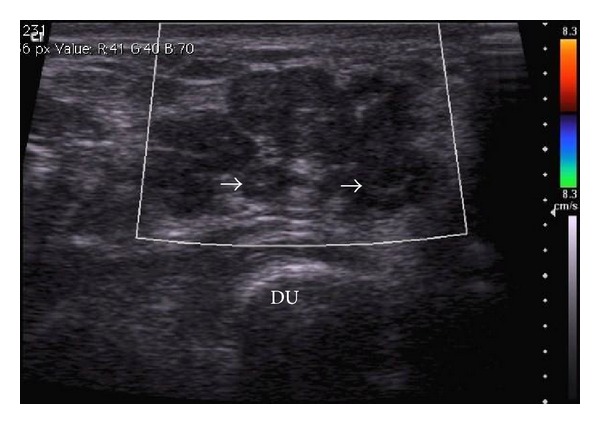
Ultrasound of the wrist—conglomerate multilobulated lesions with target appearance (white arrows): central hyperechogenicity with hypoechoic periphery. No vascularity noted on colour Doppler. DU: distal ulna.
